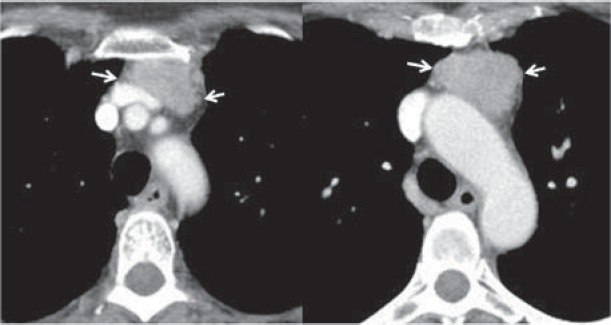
64-y/o female with myasthenia gravis; Contrast-enhanced CT scan shows slightly heterogeneous anterior mediastinal mass with irregular contour (arrows); pathologic diagnosis - type B3 thymoma
